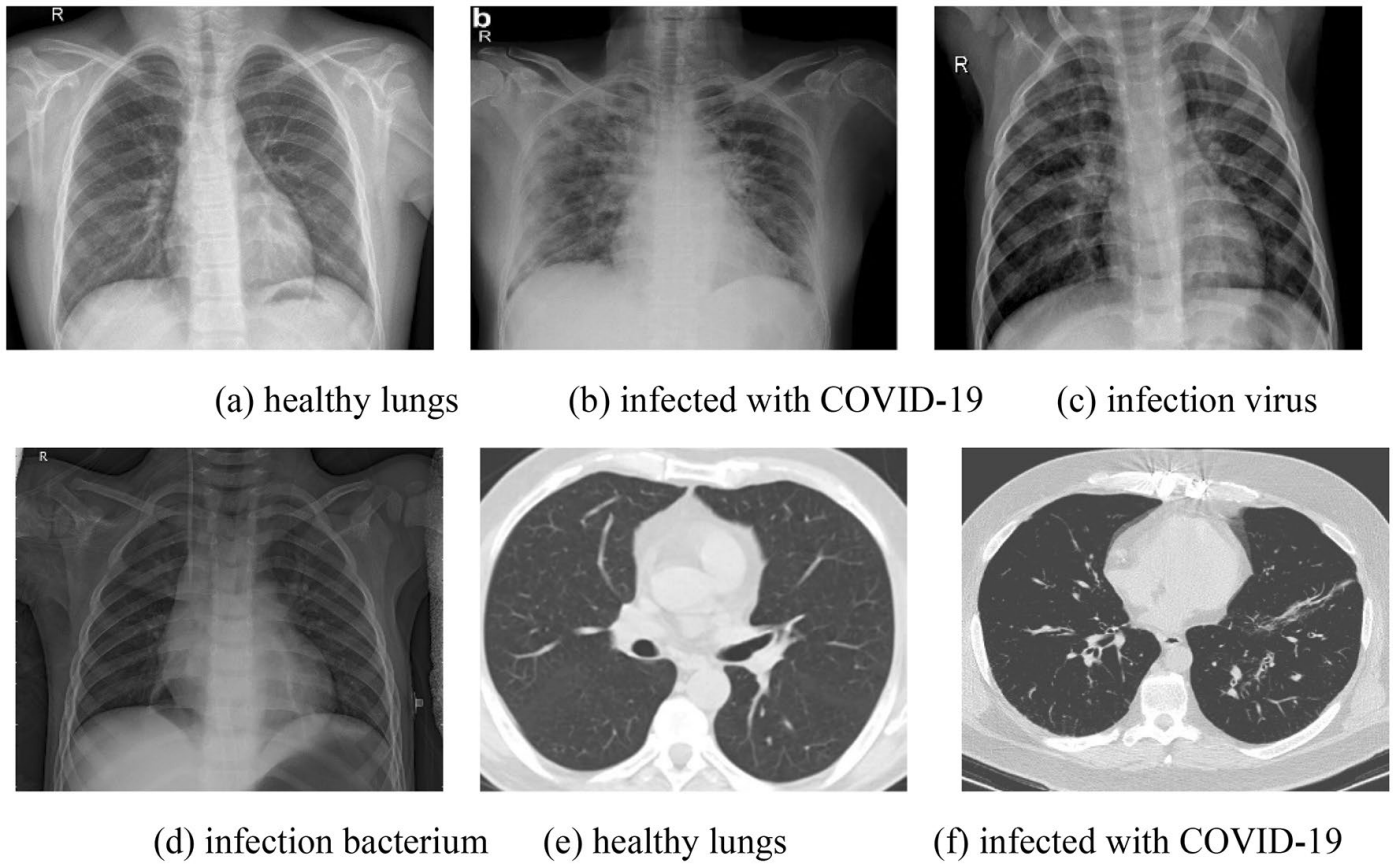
X-ray images (a–d) and CT images (e–f).
